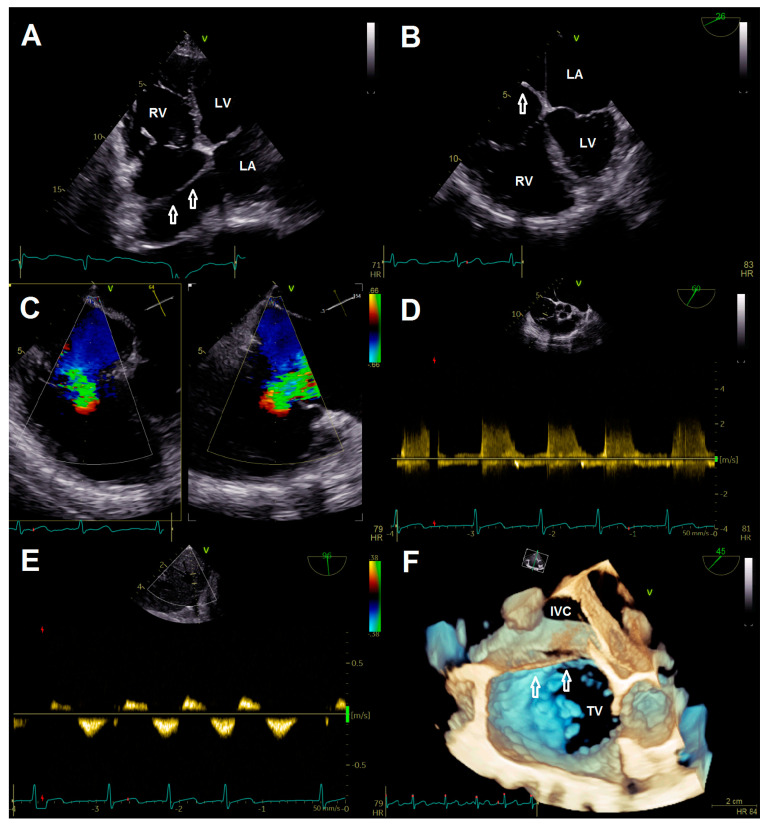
(A) Transthoracic four chamber view displaying the additional membrane in the right atrium (arrows). (B) Transesophageal four-chamber view demonstrating the membrane (arrow) resulting in a cor triatriatum. (C) Multiplane color doppler image revealing massive tricuspid regurgitation. (D) Continuous wave doppler showing no significant tricuspid gradient excluding tricuspid stenosis. (E) Flow reversal in liver veins; (F) 3-D image for visualization of access route. Abbreviations: IVC, inferior vena cava; LA, left atrium; LV, left ventricle; RV, right ventricle; TV, tricuspid valve.
